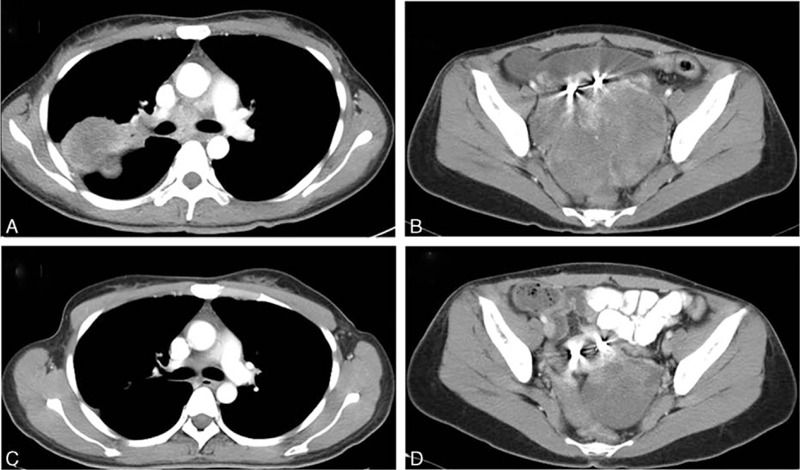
(A) Computed tomography (CT) revealed a mass at superior lobe of right lung and mediastinum lymph nodes enlargements. (B) CT revealed a mass at pelvic adnexal area. (C) CT revealed an excellent tumor response in the right lung after 2 months of treatment with crizotinib. (D) CT revealed an excellent tumor response in pelvic adnexal area after 2 months of treatment with crizotinib.
